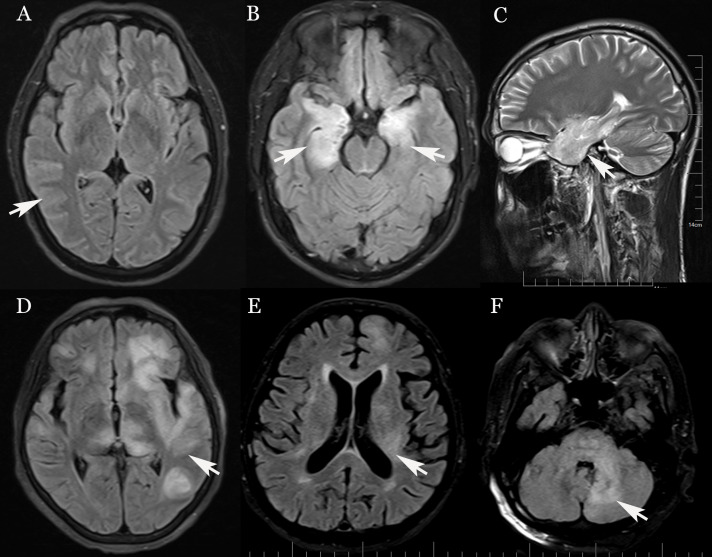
Examples of brain magnetic resonance imaging (MRI) findings of patients. Instructions for brain MRI imaging. All images shown are brain axial fluid-attenuated inversion recovery (FLAIR) images. (A) The bilateral hyperintensities in the temporal lobe cortex and insula. Bilateral medial temporal lobe and hippocampal swelling with high signal intensity on FLAIR imaging in (B, C) (sagittal sequences). (D) Multifocal hyperintensities in both frontal and temporal lobes, basal ganglia region, and left occipital lobe. (E) The Left frontal lobe, periventricular abnormal signaling. (F) High signal intensity in left cerebellar hemisphere.
